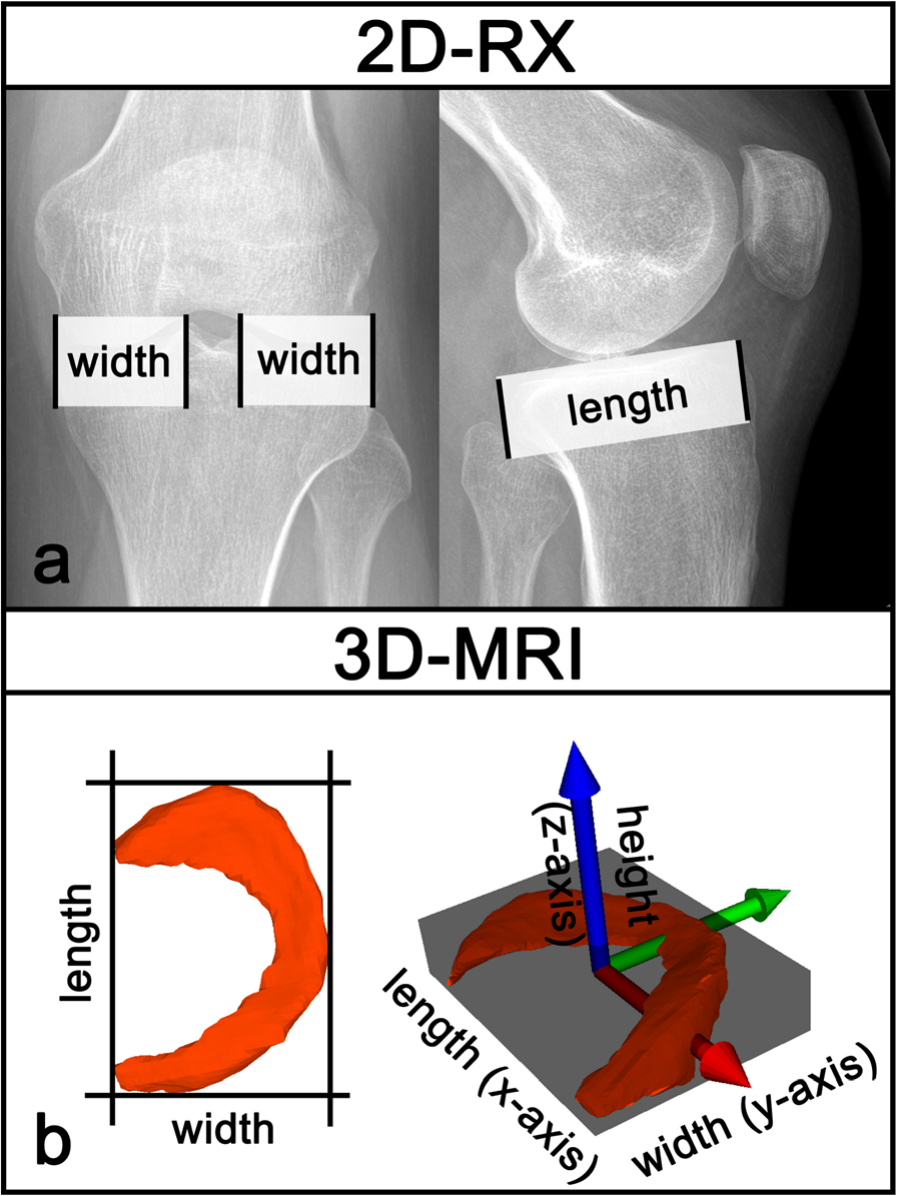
Measurement meniscus dimensions in 2D-RX, 2D-MRI and 3D-MRI. a*2D-RX: Meniscus length = Distance between the anterior surface of the tibia above the tuberosity to the posterior margin of the tibial plateau, perpendicular to the joint line. Multiplication by 0.8 for medial and 0.7 for lateral meniscus. Meniscus width = Distance from the margin of the tibial metaphysis to the medial, respectively lateral tibial eminences, perpendicular to the joint line.*b*3D-MRI: A best fitting three-dimensional box was placed around the meniscus. X-axis was parallel to a line between anterior and posterior meniscus root. X-axis = meniscus length, Y-axis = meniscus width, Z-axis = meniscus height*
